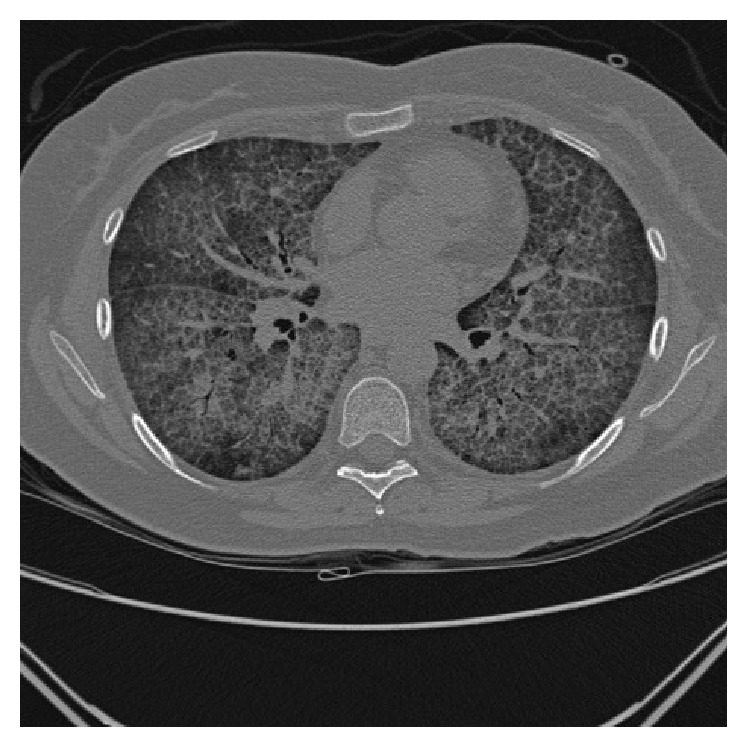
CT of the thorax depicting diffuse bilateral ground-glass opacities with intralobular septal thickening; the findings are reported by the radiologist to be a crazy paving pattern.
